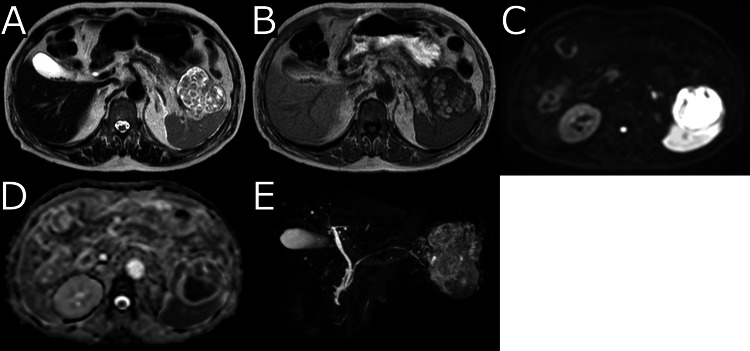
Magnetic resonance imaging Multiple subcentimeter nodules within the cyst, exhibiting heterogeneous intermediate intensity on T2-weighted image (A) and heterogeneous high intensity on T1-weighted image (B). Diffusion-weighted image demonstrated diffusion restriction (C), with an apparent diffusion coefficient value of approximately 0.400 ×10-3mm2/s (D). Magnetic resonance cholangiopancreatography demonstrated no apparent communication with the main pancreatic duct (E).
